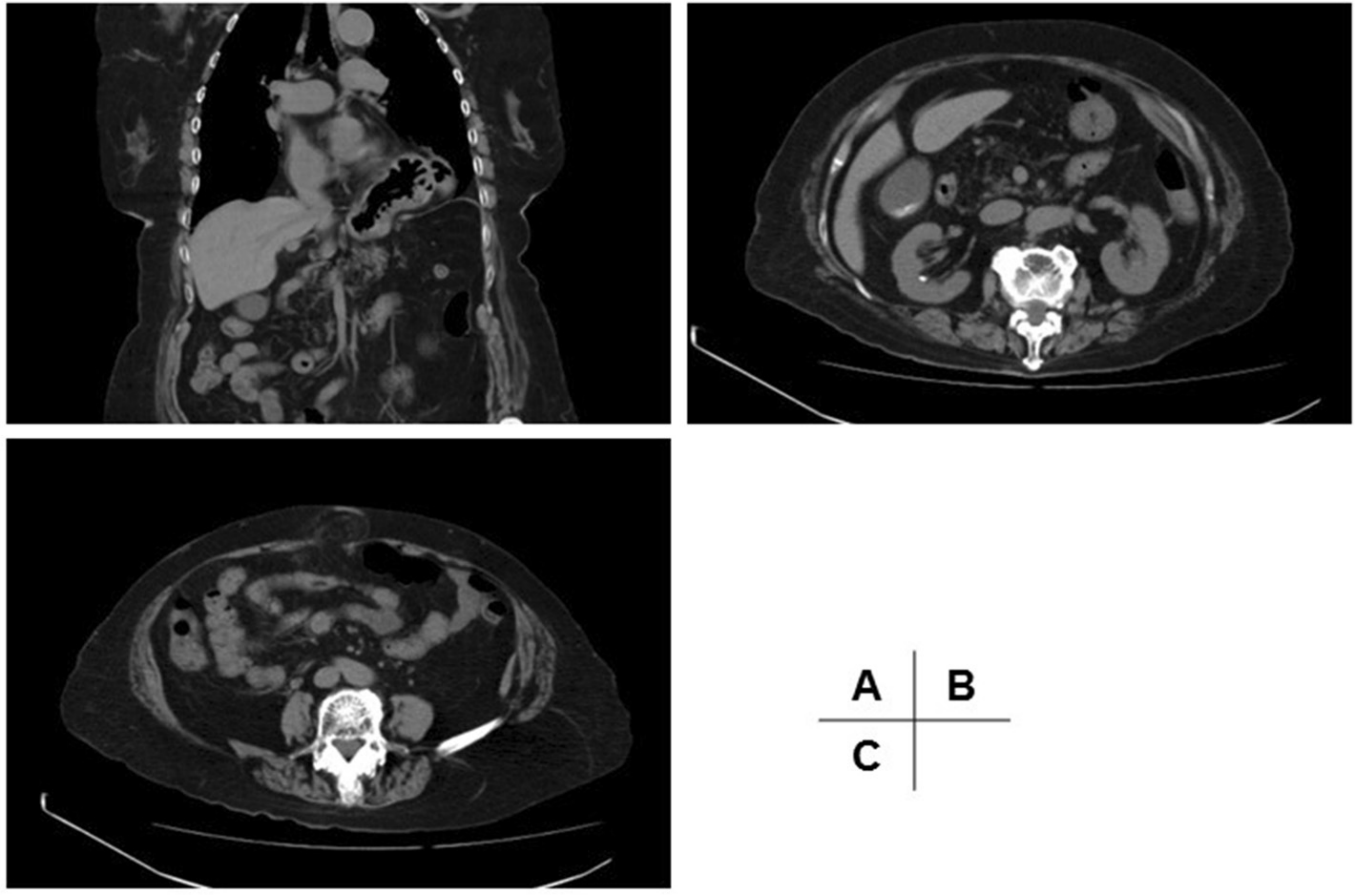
Images of Case 1. a CT revealed a hiatal hernia, through which the fundus of the stomach slid into the intrathoracic cavity. b CT revealed the presence of cholelithiasis. c Abdominal CT showed the umbilical hernia with accompanying prolapse of intestinal fat tissue
